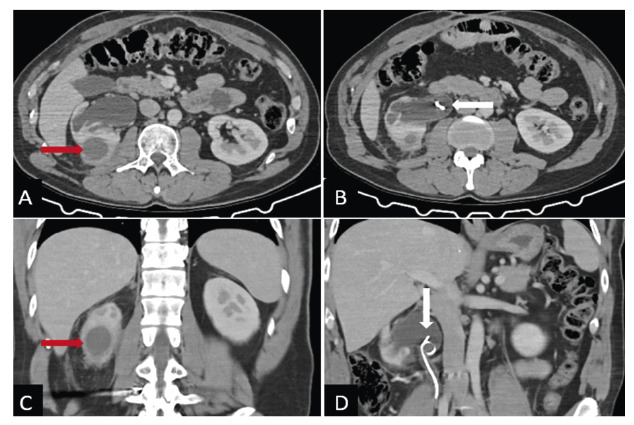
Axial (A-B) and coronal (C-D) plane enhanced abdomen computed tomography shows a well-defined collection resembling an abscess in the right kidney. The collection has a diameter of 3 cm at its lower pole (red arrows). Additionally, grade 3 hydronephrosis and double-J stent are observed in the right kidney (white arrows).
